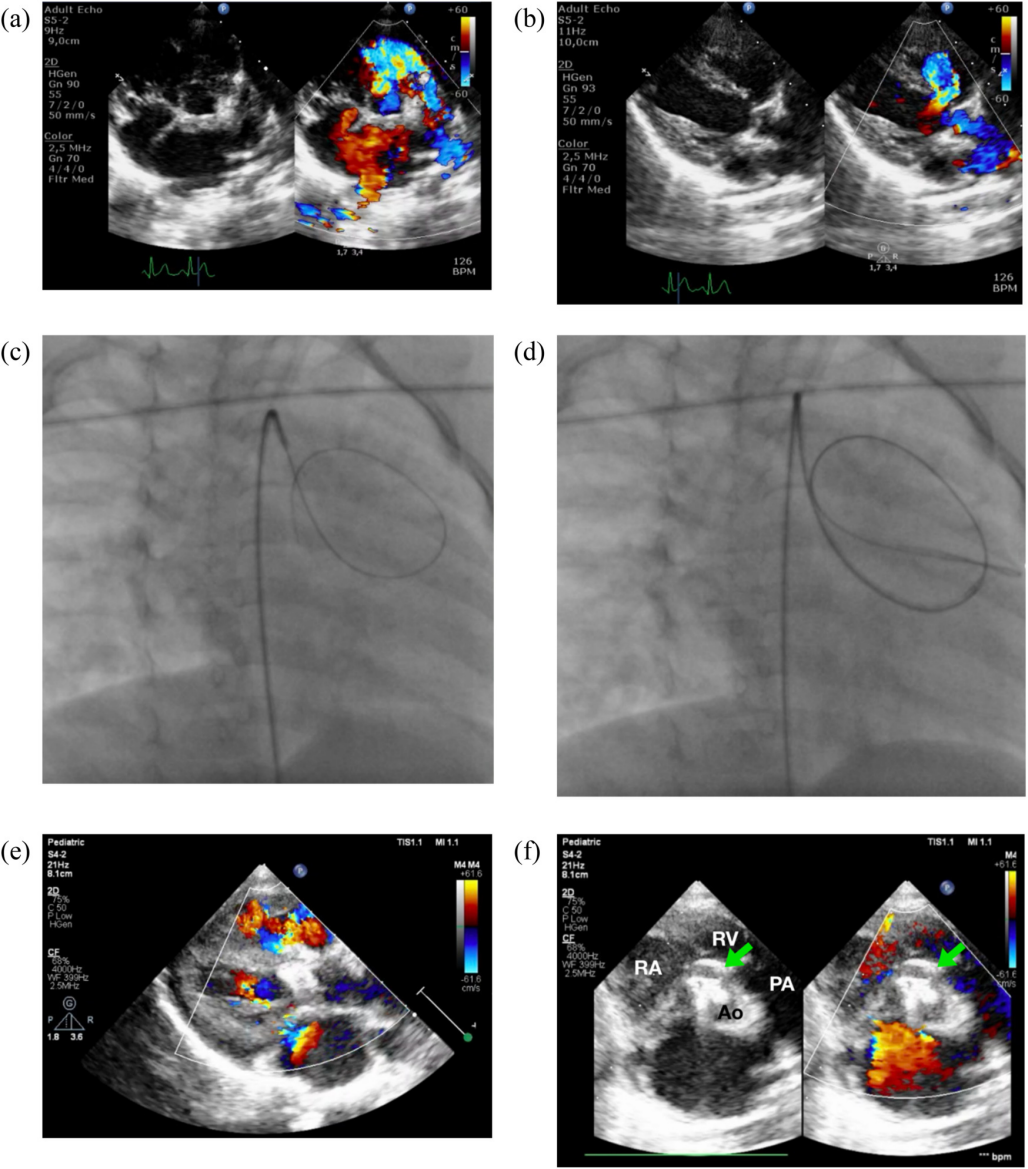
Zero-contrast closure of a doubly-committed subarterial VSD. Transthoracic echocardiography revealed a DCSA VSD in both the parasternal short-axis/PSAX (a) and long-axis/PLAX (b) views. Simultaneous fluoroscopy with the view of RAO 30/CRAN 0 (c,d) and transthoracic echocardiography was employed to cross the VSD by using 3.5/5F JR guiding catheter and 0.035″ soft hydrophilic exchange wire. Following device deployment, imaging confirmed proper device placement without evidence of aortic or tricuspid regurgitation (e). (f) PSAX view at aortic level confirmed device edge is touching the pulmonary valve, but no aortic regurgitation was observed. RA, right atrium; RV, right ventricle; PA, pulmonary artery; Ao, aorta.
